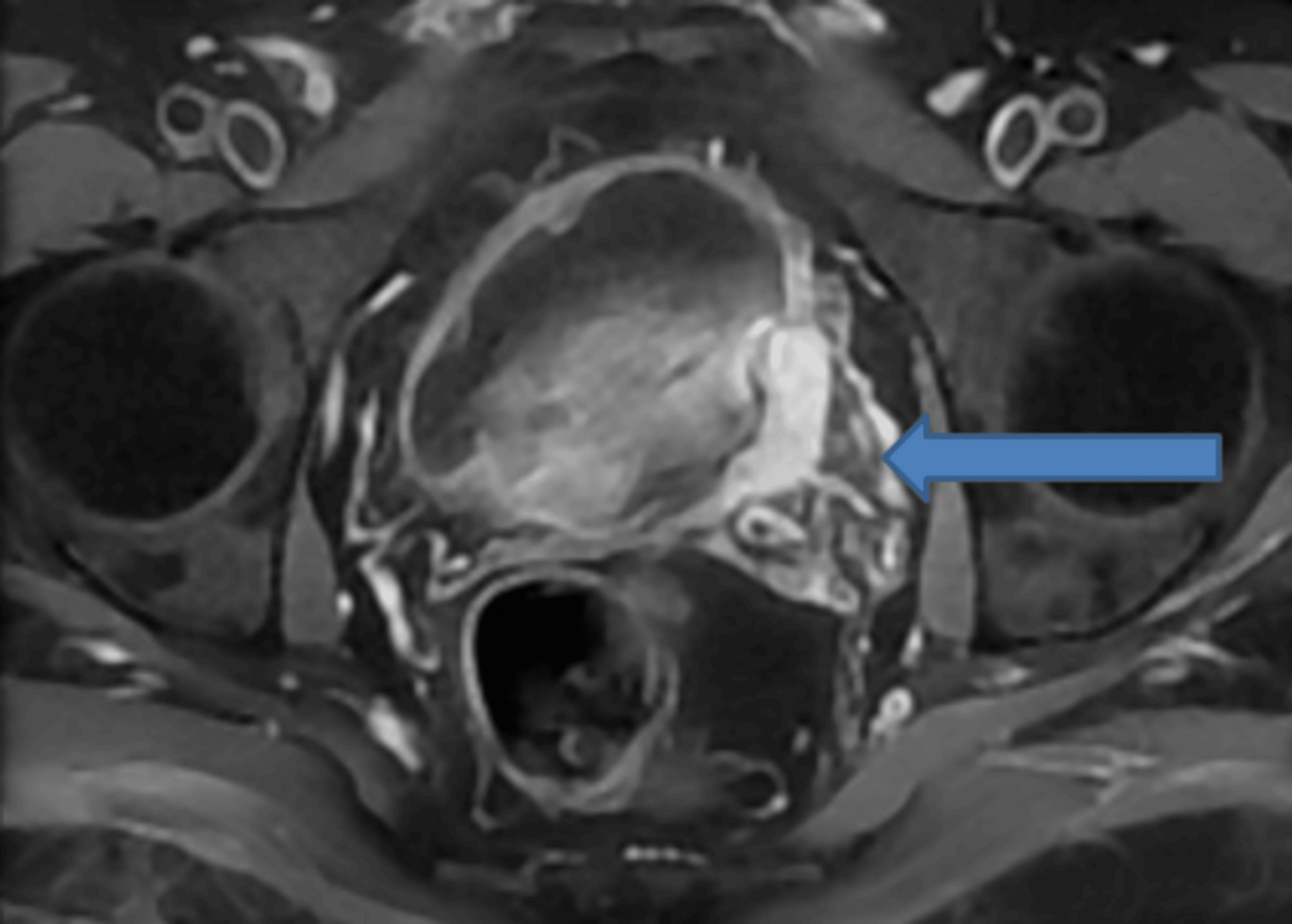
Contrast-enhanced T1-weighted MRI of the abdomen (axial view) showing enhancing soft tissue thickening along the left lateral urinary bladder wall (blue arrow) consistent with biopsy-proven transitional cell carcinoma.
